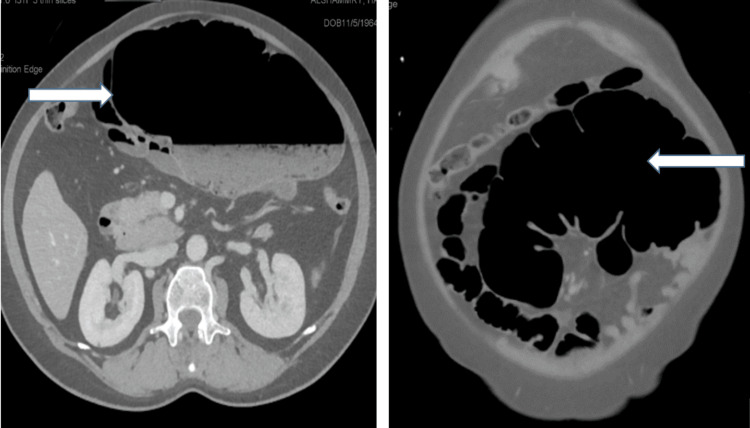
Tow (axial views) of the CT scan abdomen showing marked distension of the cecum measuring about 12 mm and extending from the right lower quadrant to the epigastrium (white arrows).
